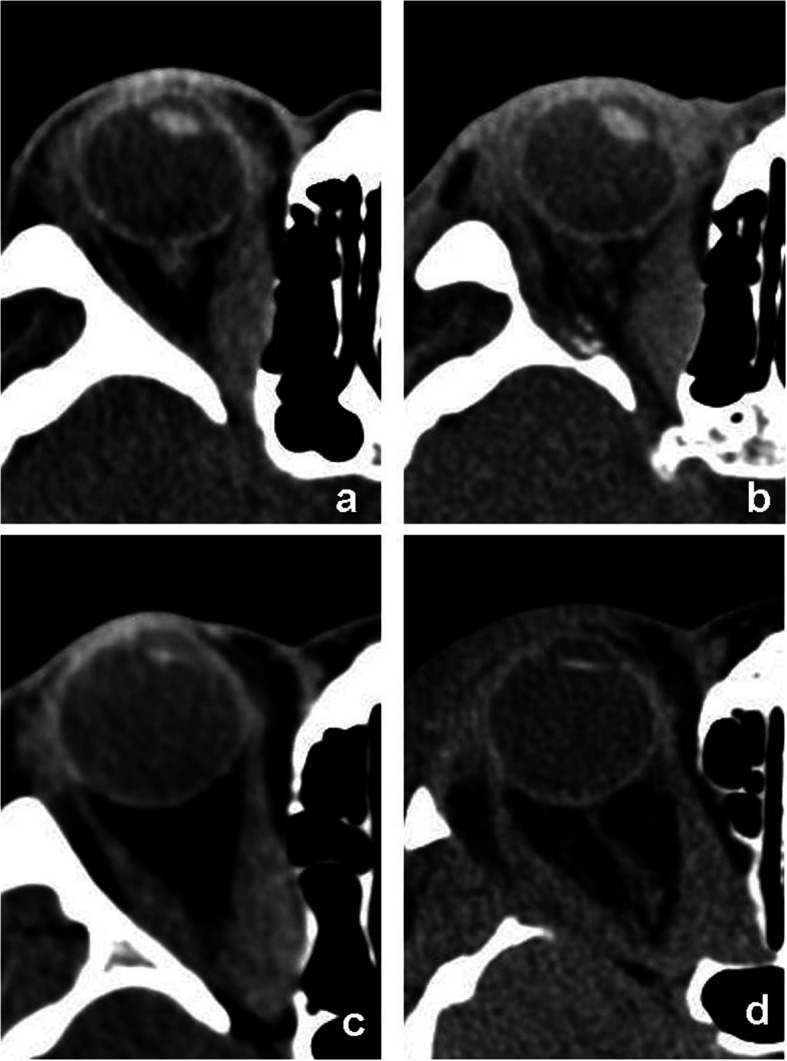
Pre- and postoperative CT images of the patients who underwent orbital decompression. a-b A subject who underwent 1-wall decompression had an obvious increase in esotropia, the medial rectus became thicker after surgery. c-d A subject who underwent 2-wall decompression had no worsening of strabismus. The position of the medial rectus changed but the thickness did not change much
